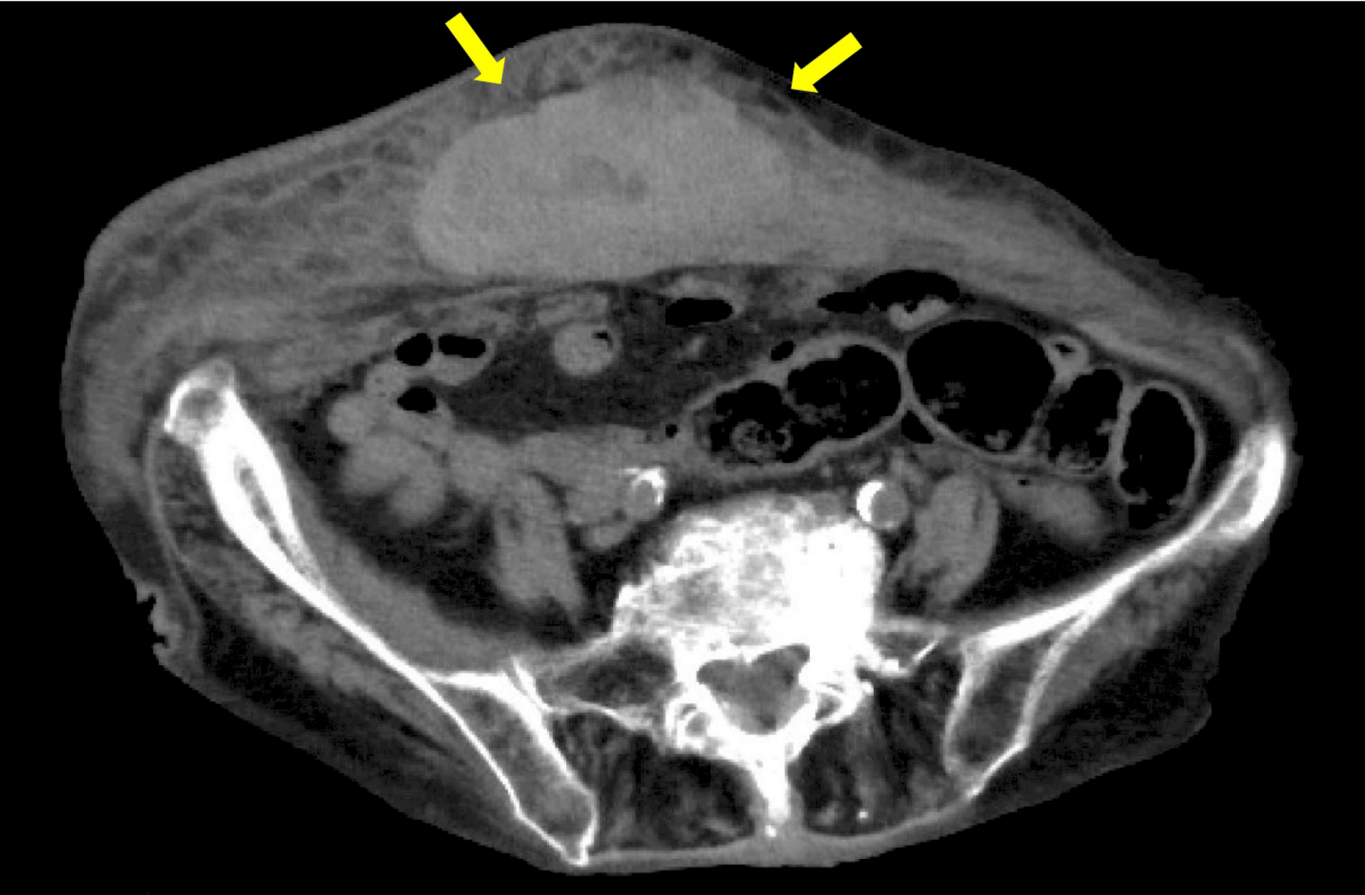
CT scan showing a rectus sheath hematoma on admission.
